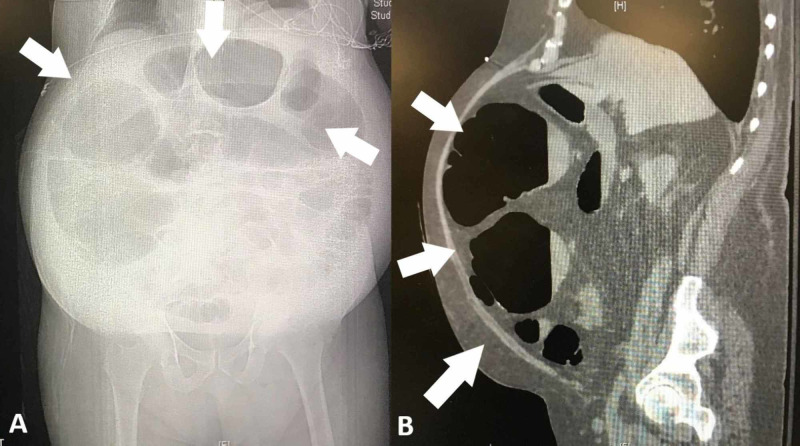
Abdominal X-ray revealing moderate to severe dilatation of the colon (A). CT scan with oral contrast showing a severe dilatation of colon (B).
